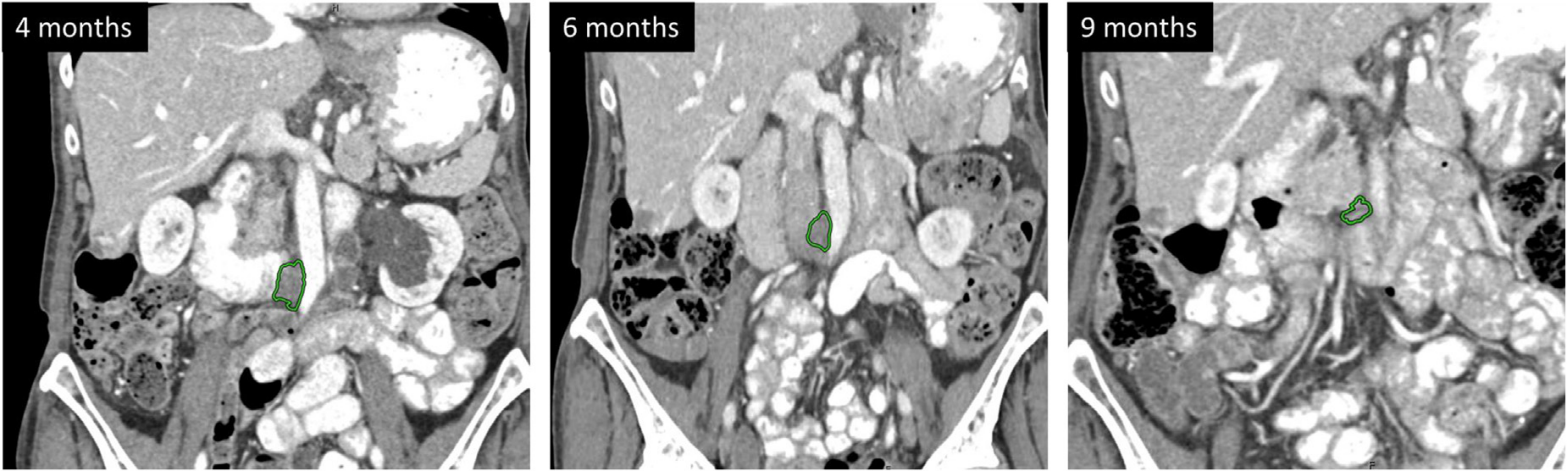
Serial imaging demonstrating treatment response in aortocaval lymph node (LN1) and complete resorption of the hydrogel spacer following stereotactic body radiation therapy (SBRT). Coronal intravenous (IV) and oral contrast-enhanced abdominal computed tomography (CT) images demonstrate a progressive decrease in the size of the radiated aortocaval lymph node, consistent with treatment response. The lymph node measured 18 × 19 × 35 mm pretreatment (Fig. 1A), with subsequent reductions to 9 × 12 × 19 mm at 4 months, 8 × 12 × 11 mm at 6 months, and 7 × 10 × 8 mm at 9 months posttreatment. Additionally, the previously placed SpaceOAR is no longer detectable on posttreatment scans after 4 months, indicating complete resorption.
